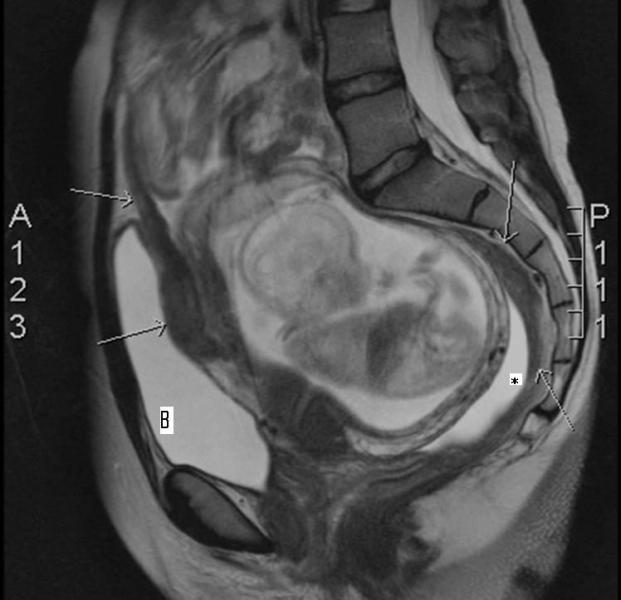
Magnetic resonance imaging findings of diffuse peritoneal involvement by a poorly differentiated sarcoma. Sagittal T2-weighted turbo spin-echo magnetic resonance image (repetition time msec/echo time msec = 8440/136) shows diffuse sheetlike and nodular thickening of the peritoneal surfaces (arrows). Note also a moderate amount of ascites (asterisk). Bladder (B).
